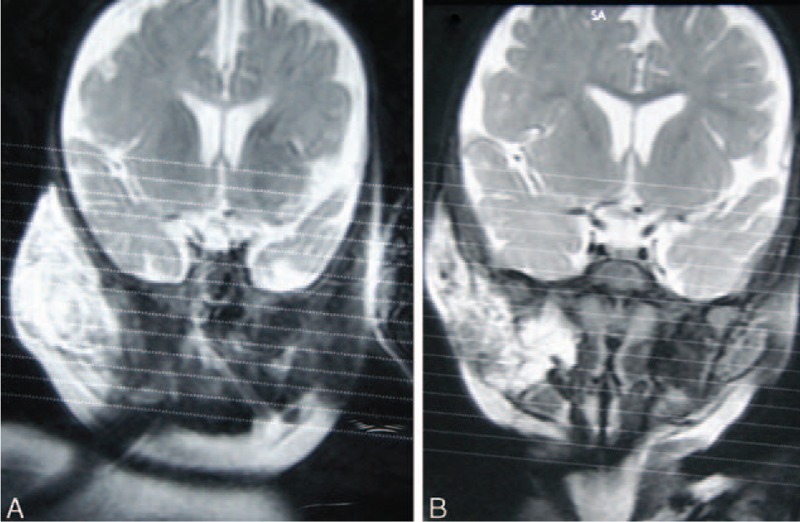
T2-weighted magnetic resonance imaging of a male patient with deep microcysitic lymphatic malformations located in the right cheek. (A) 6 months of age; before starting sclerotherapy with pingyangmycin; (B) 12 months of age; after 3 injections of pingyangmycin, obvious decrease in size and signal of the lesion was shown in T2-weighted magnetic resonance imaging.
